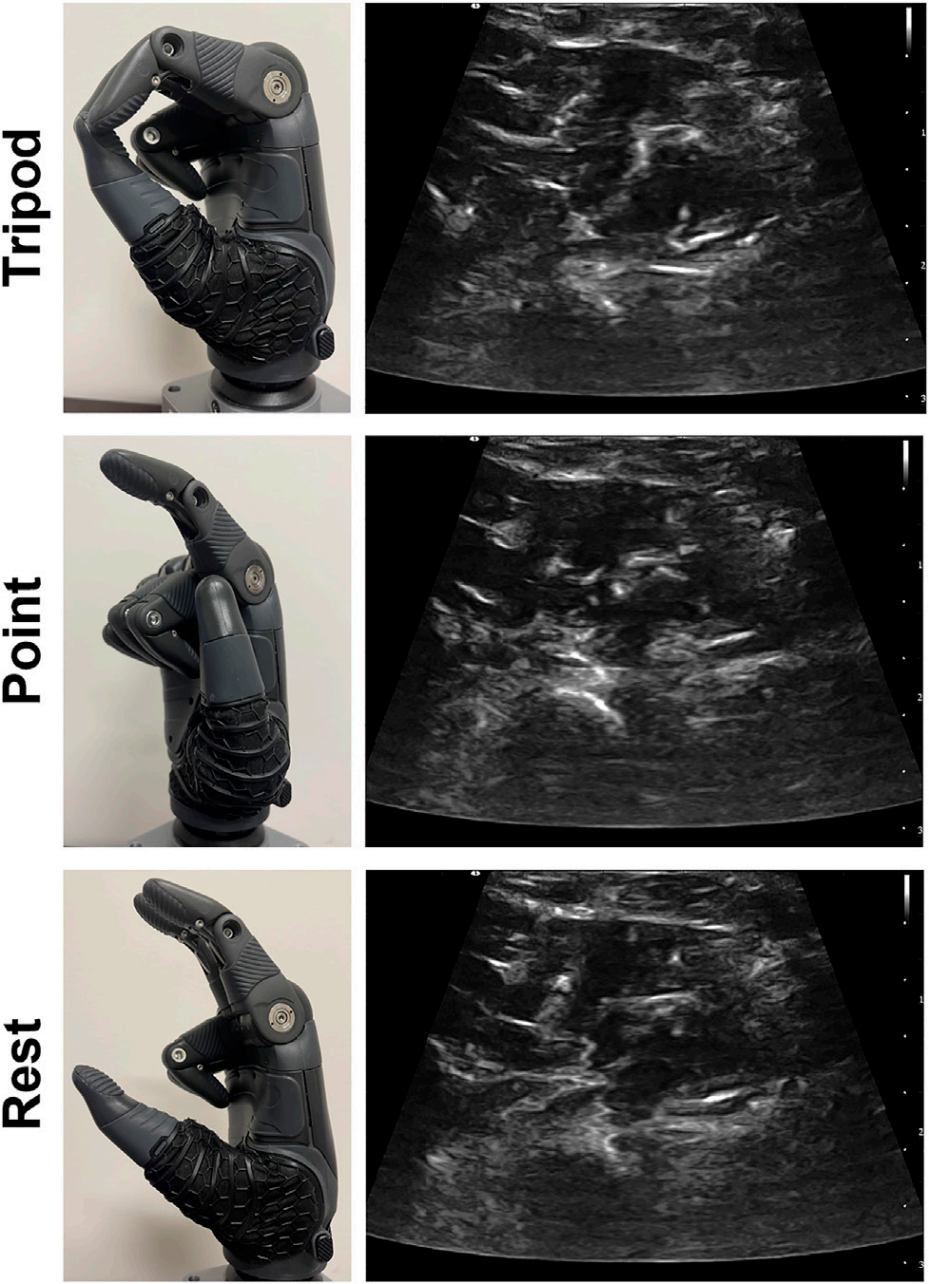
The TASKA hand was configured to include *tripod* grasp, index finger *point*, and *rest*. Each grasp was initiated in response to a different muscle activation pattern, including wrist flexion for *tripod*, wrist extension for *point*, and a relaxed muscle state for *rest*. Representative ultrasound images are included for each muscle activation pattern.
